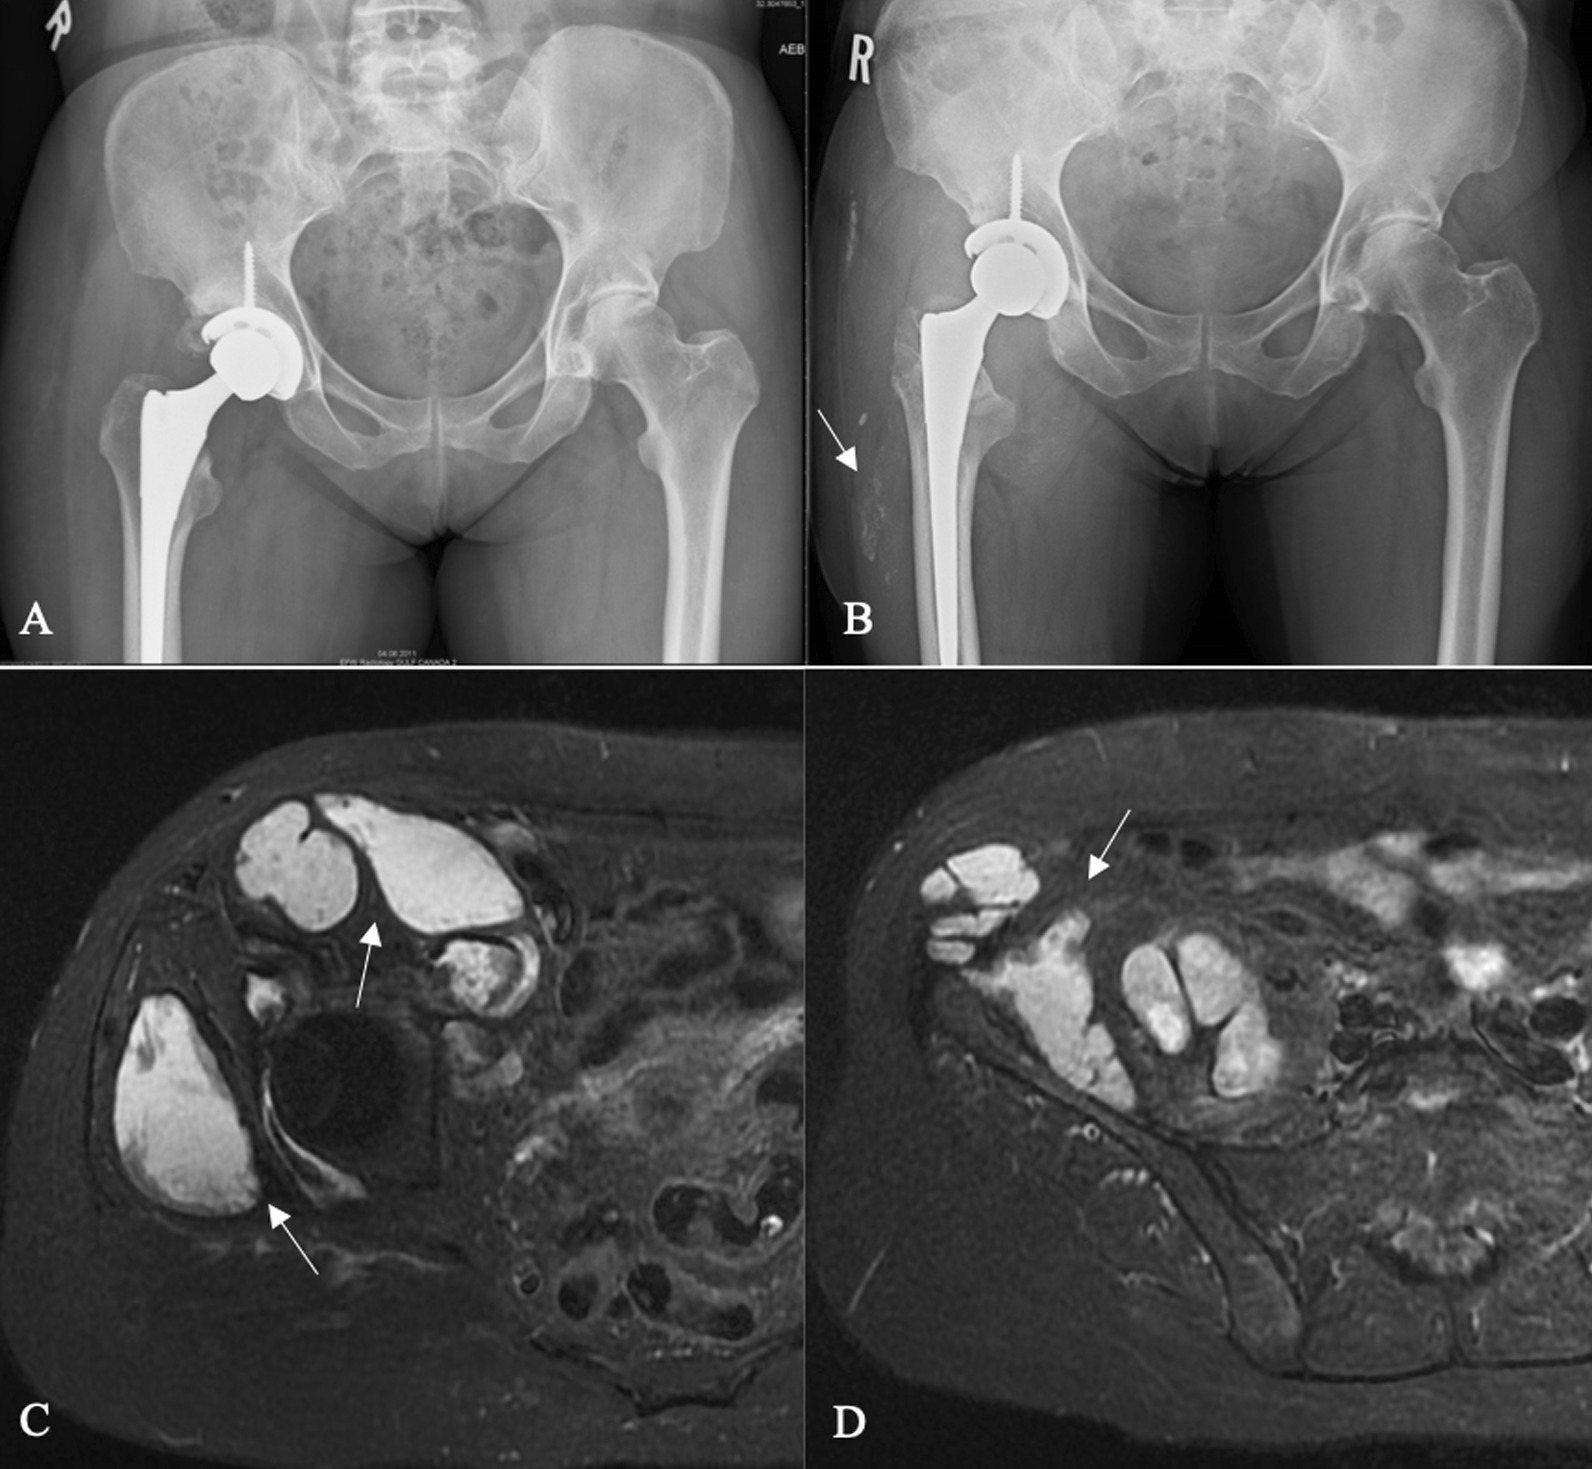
Select preoperative images: A anteroposterior (AP) pelvis radiograph following index right THA; B AP pelvis radiograph prior to revision demonstrating soft-tissue shadowing and mineralization surrounding the right hip and proximal femur (arrow); C, D axial MRI slices revealing significant pseudotumor extension (arrows)
